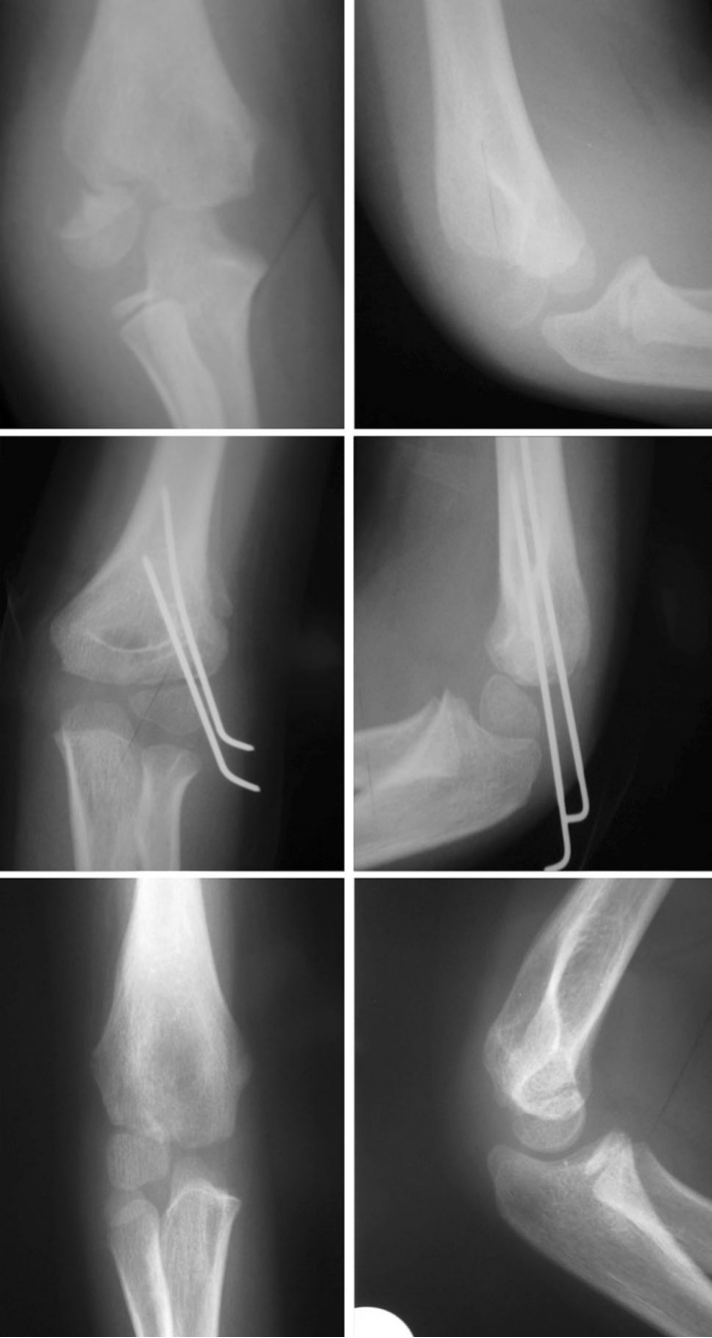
6-year-old male, fracture healed at 5 weeks, X-ray at 1 year demonstrates healing without lateral hypertrophy
